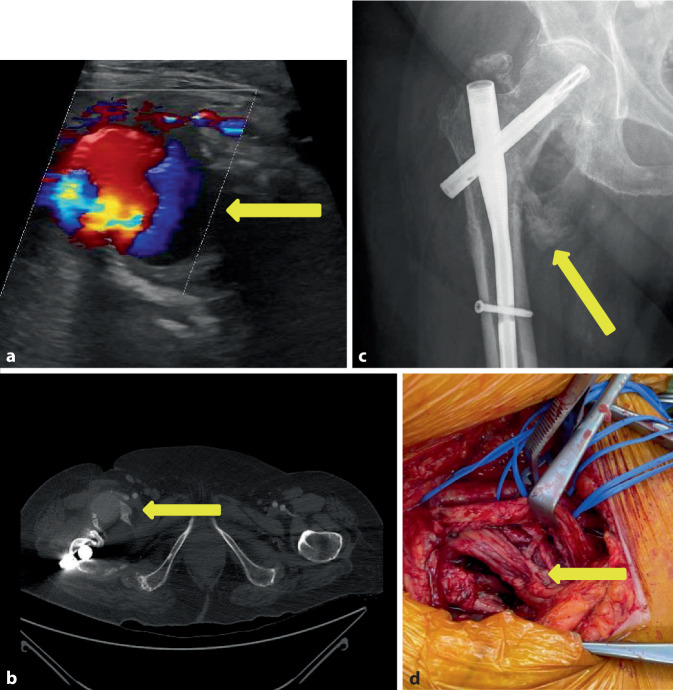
Fall 2: Pseudoaneurysma der A. profunda femoris rechts. a Farbkodierte Duplexsonographie des Pseudoaneurysmas mit typischem Farbwechsel (*gelber Pfeil*), b CT-Angiographie mit Nachweis des Pseudoaneurysmas der rechten Inguinalregion (*gelber Pfeil*), c Nativröntgen der mit einem PFN-Nagel versorgten pertrochantären Fraktur mit zirkulärer Verkalkung (*gelber Pfeil*), d intraoperative Darstellung der Pseudoaneurysmahöhle und des verletzten Profundaastes (*gelber Pfeil*)
